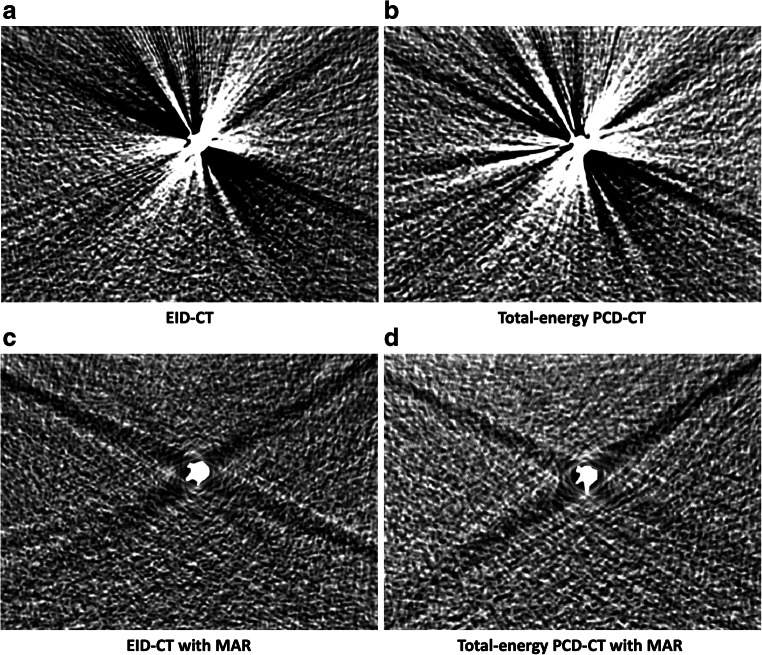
Representative images of the experimental in vitro model, resembling a coil-embolized aneurysm of the cerebral arteries, in energy-integrating computed tomography (EID-CT) and photon-counting detector computed tomography (PCD-CT, total-energy images) before (a: EID-CT; b: total-energy PCD-CT) and after metal artifact reduction (MAR; c: EID-CT; d: total-energy PCD-CT). Note the profound reduction of the coil-related artifacts. All images are provided in a standard CT brain imaging window (width: 40 Hounsfield units (HU) and level/center: 80 HU)
